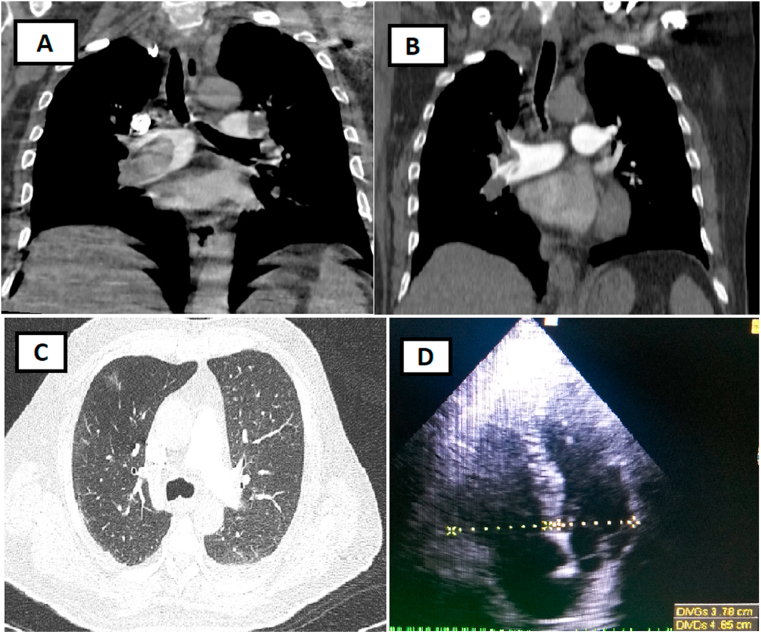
CT pulmonary angiography showing A: Pulmonary artery thrombosis extended to its right and left branches with total obstruction of their lumens B: Residual right lobar and segmental PE with left segmental PE 48 hours after thrombolytic therapy. C: Bilateral peripheral ground-glass opacities classified CORADS 4. D: Apical 4 chamber view of echocardiogram showing dilated right ventricle (RV) and septal deviation due to pressure overload in RV.
